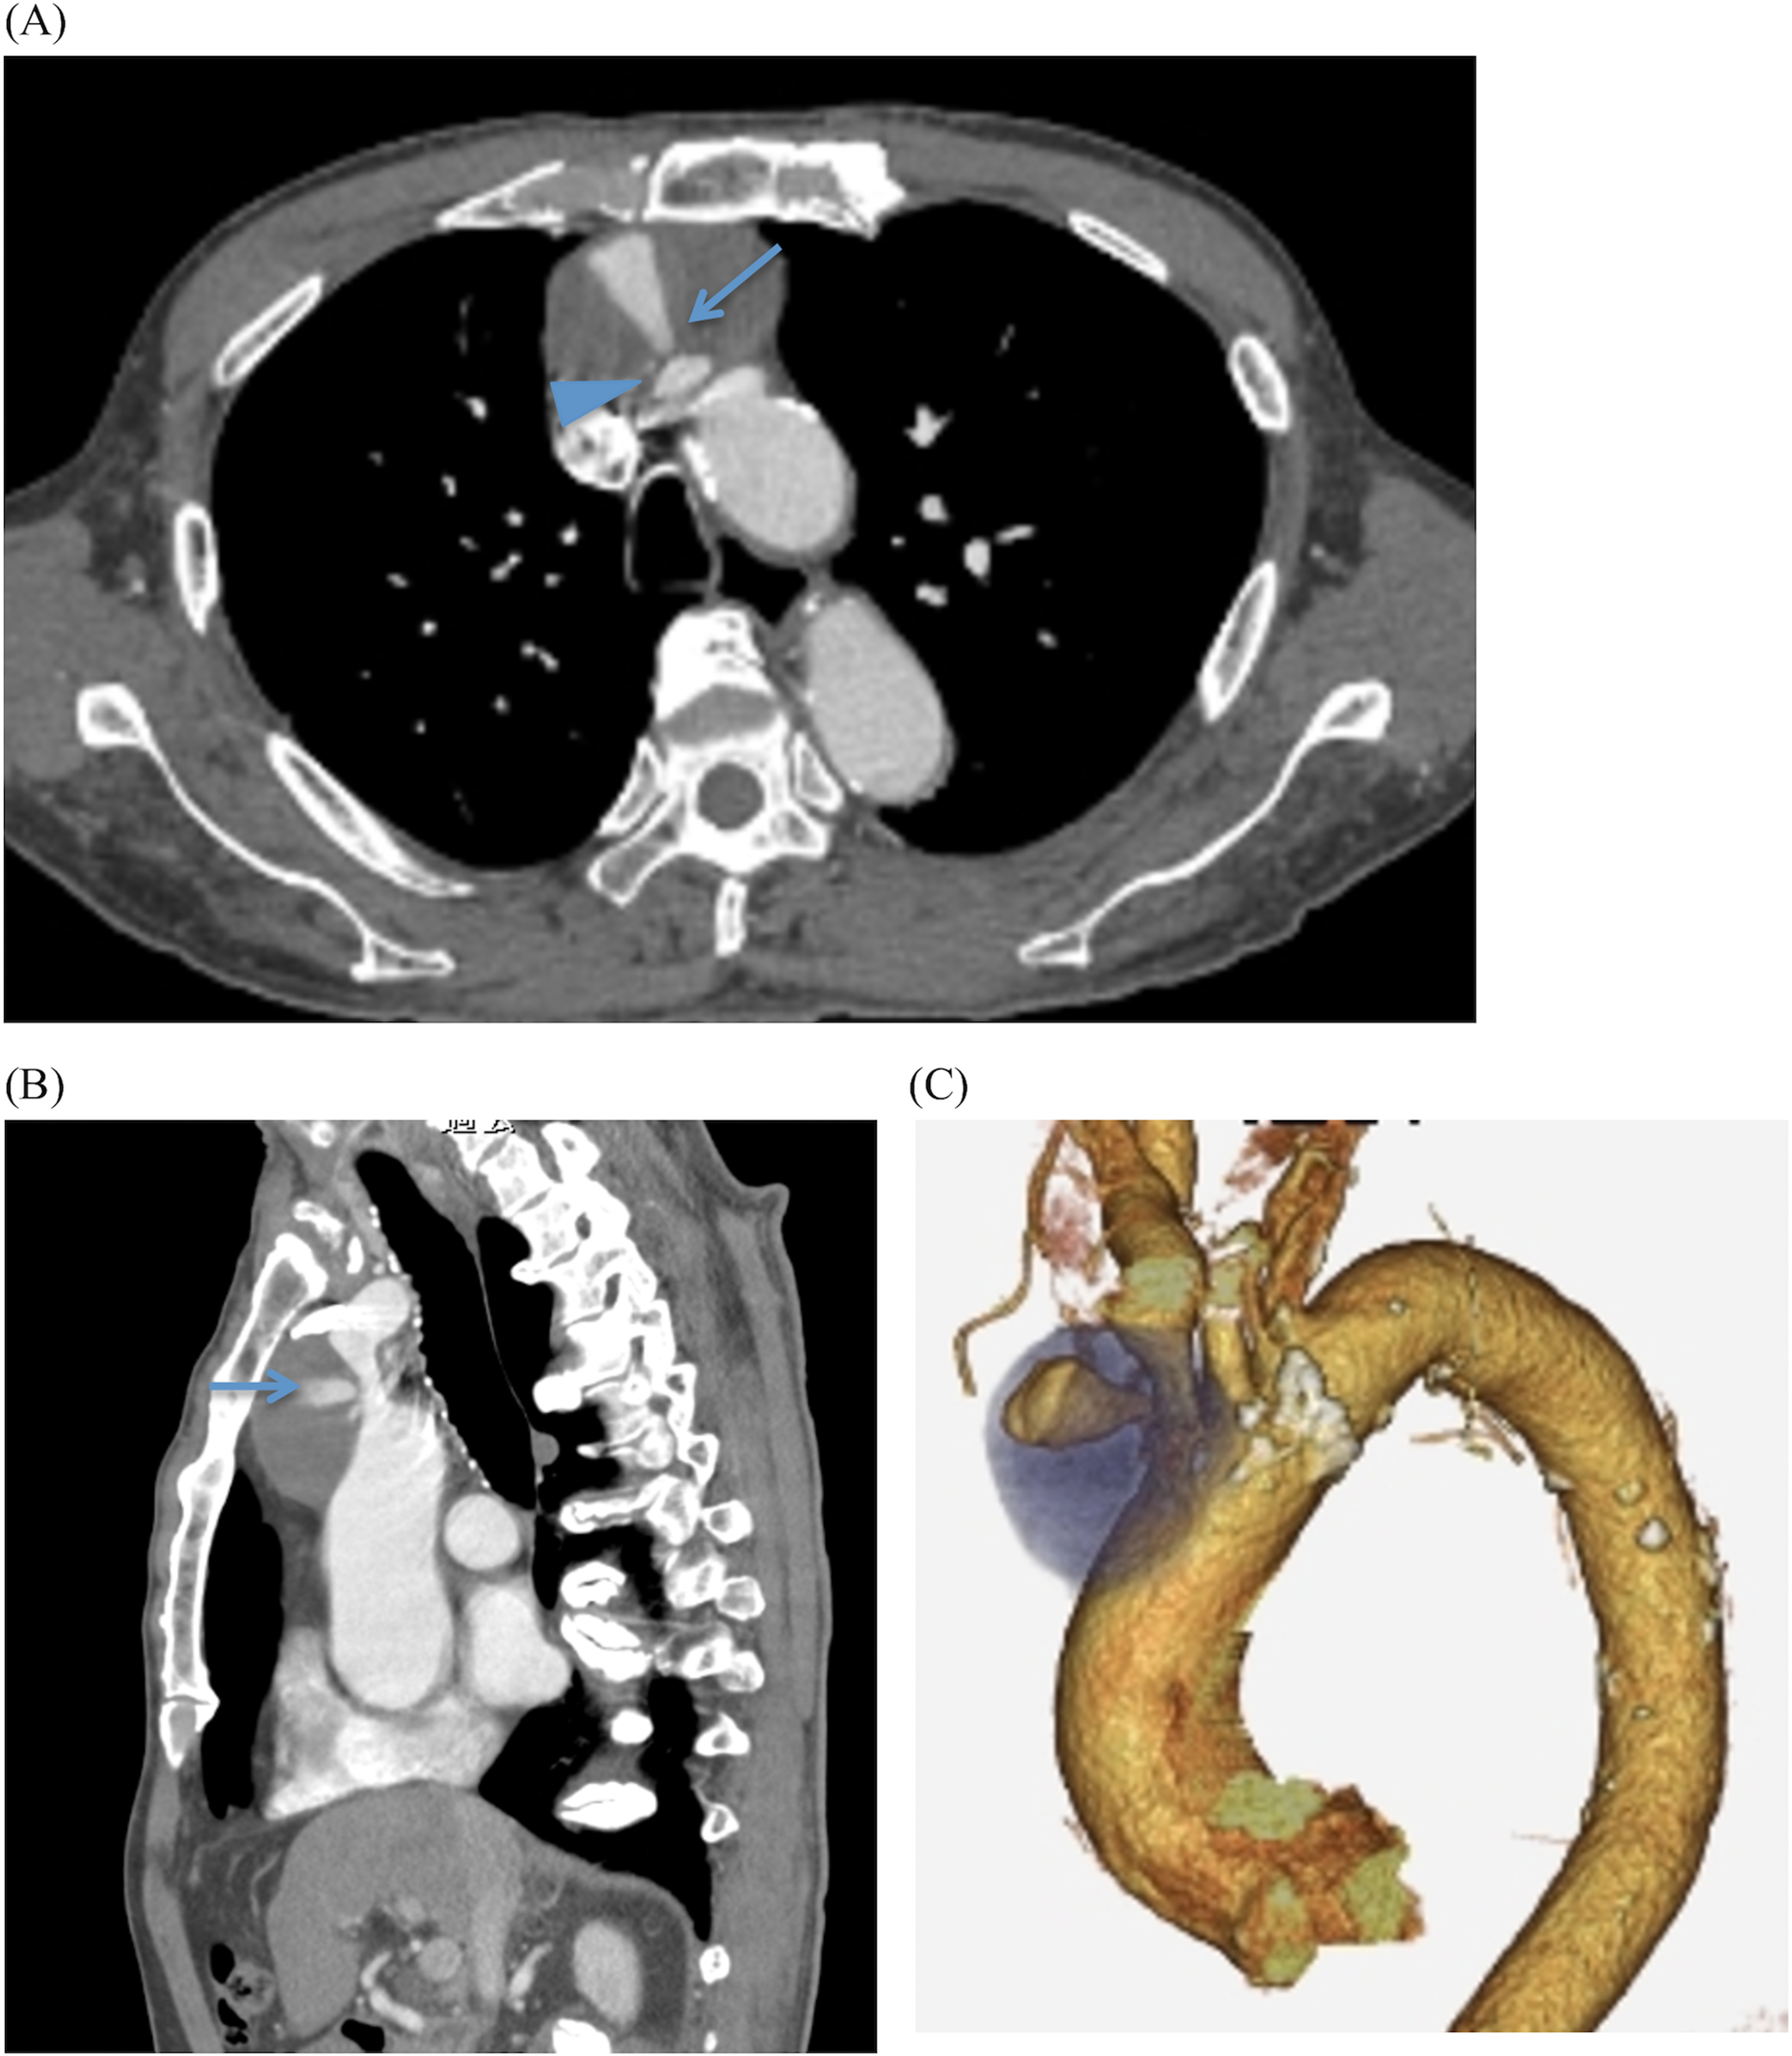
Fig. 1 Preoperative computed tomography images. (A) Coronal view showing the compressed brachiocephalic artery (arrowhead) and contrast leakage (arrow) from the brachiocephalic artery to the aneurysm. (B) Sagittal view showing the leakage point (arrow) is in the middle of the brachiocephalic artery. (C) Three-dimensional constructed image providing an overview of the aneurysm (60×57×38 mm).
